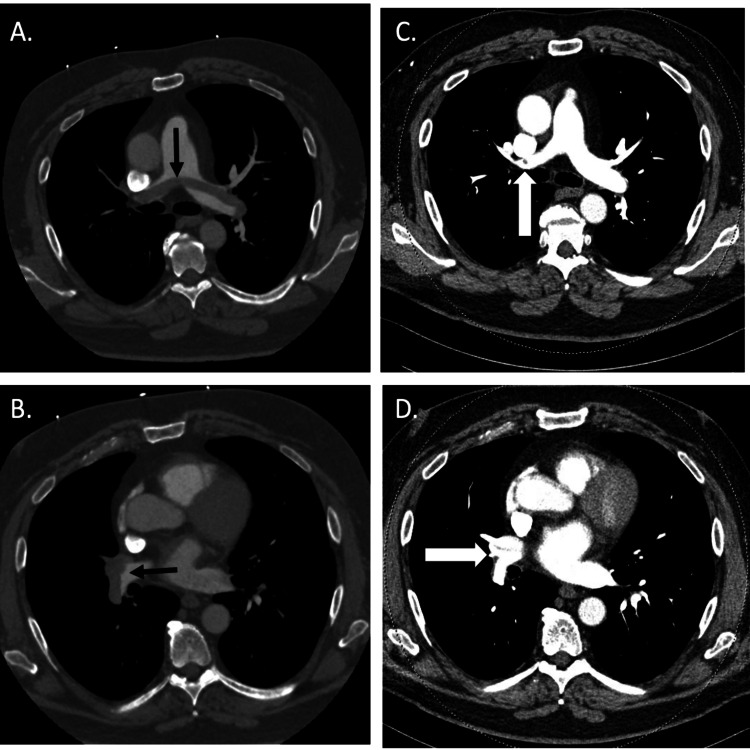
Patient's CT pulmonary angiography Representative images of initial CT pulmonary angiography (left panels) demonstrating acute pulmonary emboli (black arrows) with saddle pulmonary emboli extending into the right upper lobe (A) and right lower lobe (B) pulmonary arteries compared with three months later (right panels) demonstrating residual chronic thrombus (white arrows) at take-off of right upper lobe (C) and right lower lobe (D).
